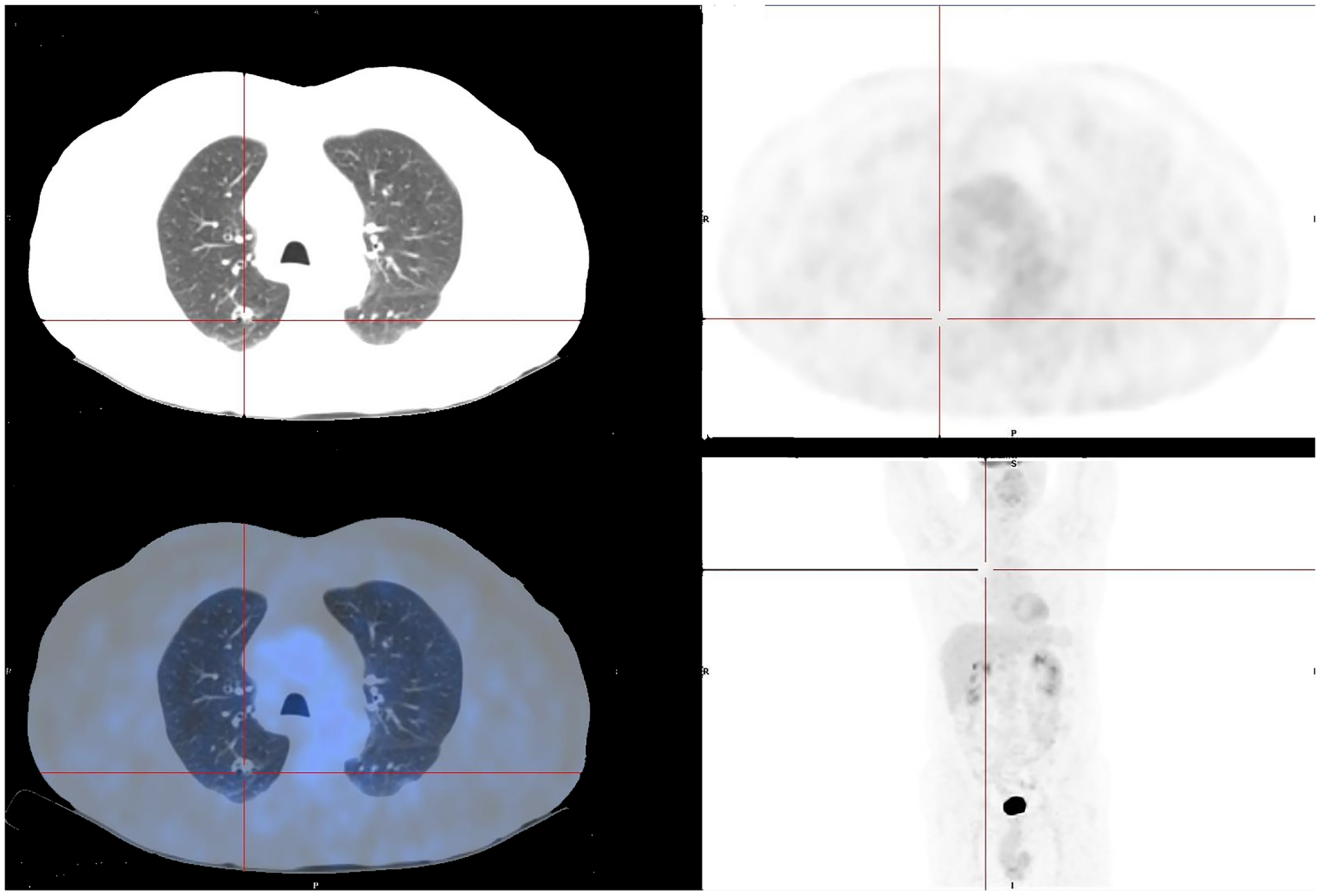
PET/CT-FDG scan one month after pembrolizumab suspension. The image shows a fibrous-cicatricial nodule with no FDG uptake in the superior right lobe.
